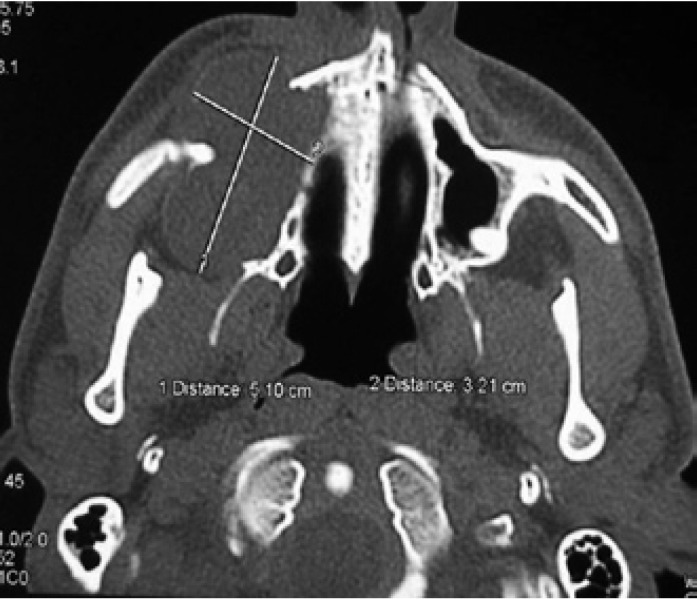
CBCT shows a large KCOT in the right maxillary sinus causing expansion of the medial and lateral sinus wall. The border of the lesion is apparent and well-circumscribed.
